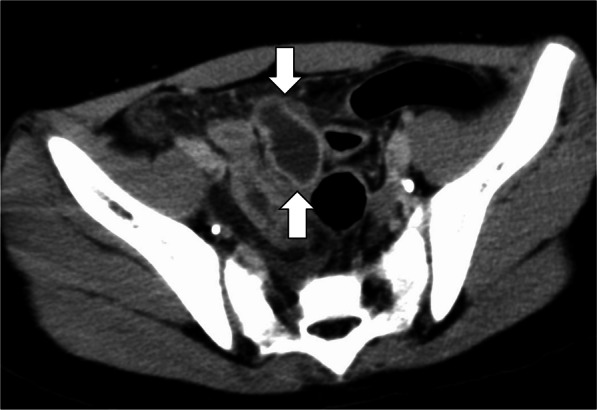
Abdominal image. Preoperative abdominal enhanced computed tomography showing an abscess in the lower abdomen (arrow), which was ultimately diagnosed as Meckel’s diverticulum
